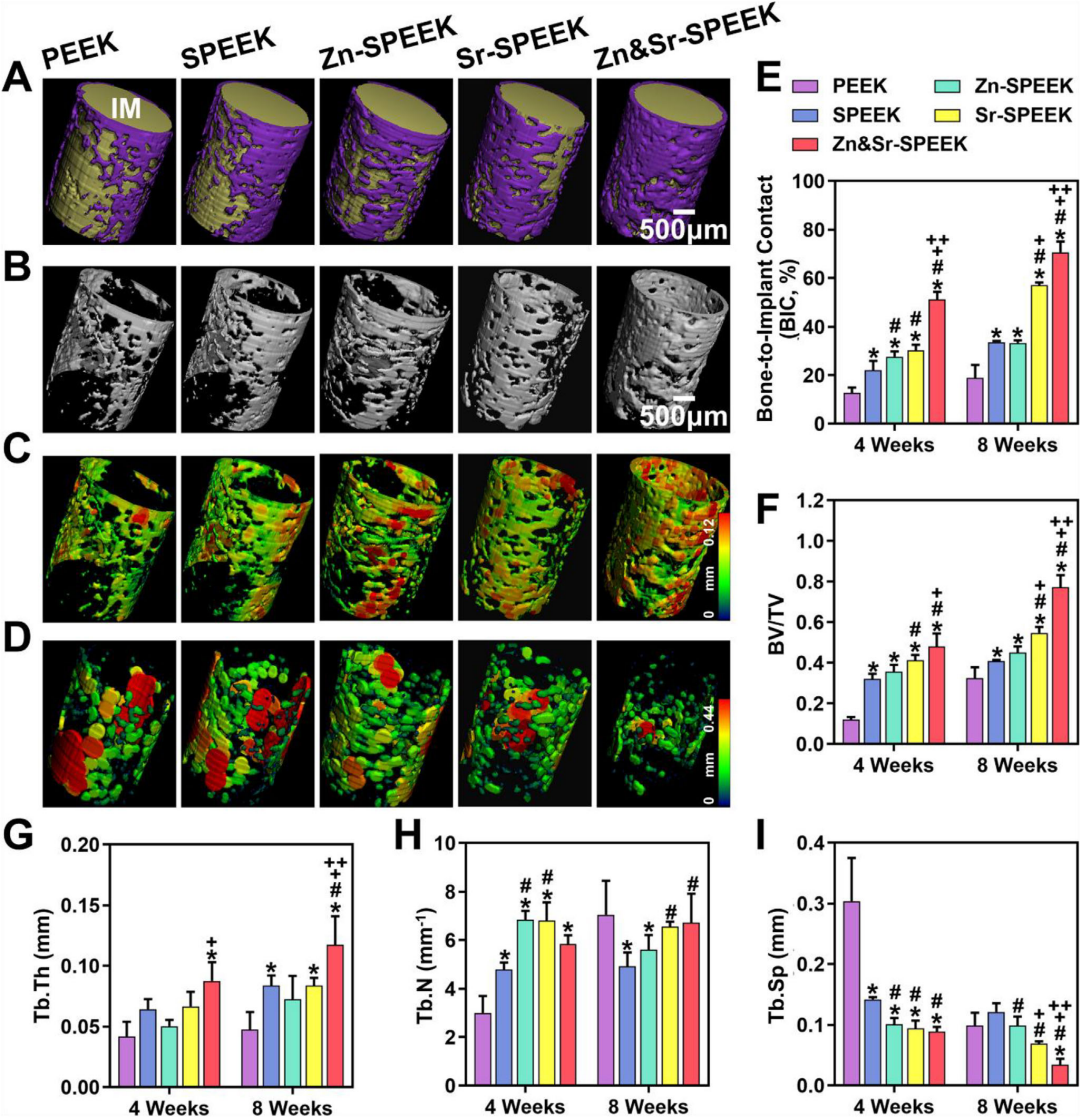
The quality and quantity of newly regenerated bone tissues surrounding the implants: the 3D reconstructed micro‐CT images of newborn bone A) with implants and B) without implants at 8 weeks, as well as E) the quantification of the bone‐implant contact value (implant (IM), yellow; new bone, purple). Representative images of C) Tb.Th and D) Tb.Sp at 8 weeks. Quantitative analysis of Micro‐CT data including F) BV/TV, G) Tb.Th, H) Tb.N, and I) Tb.Sp. (Two‐way ANOVA and Tukey's post hoc test were used; *n* = 4; *, #, +, and ++ represent *p* < 0.05 when compared with PEEK, SPEEK, Zn‐SPEEK, Sr‐SPEEK, respectively.)
